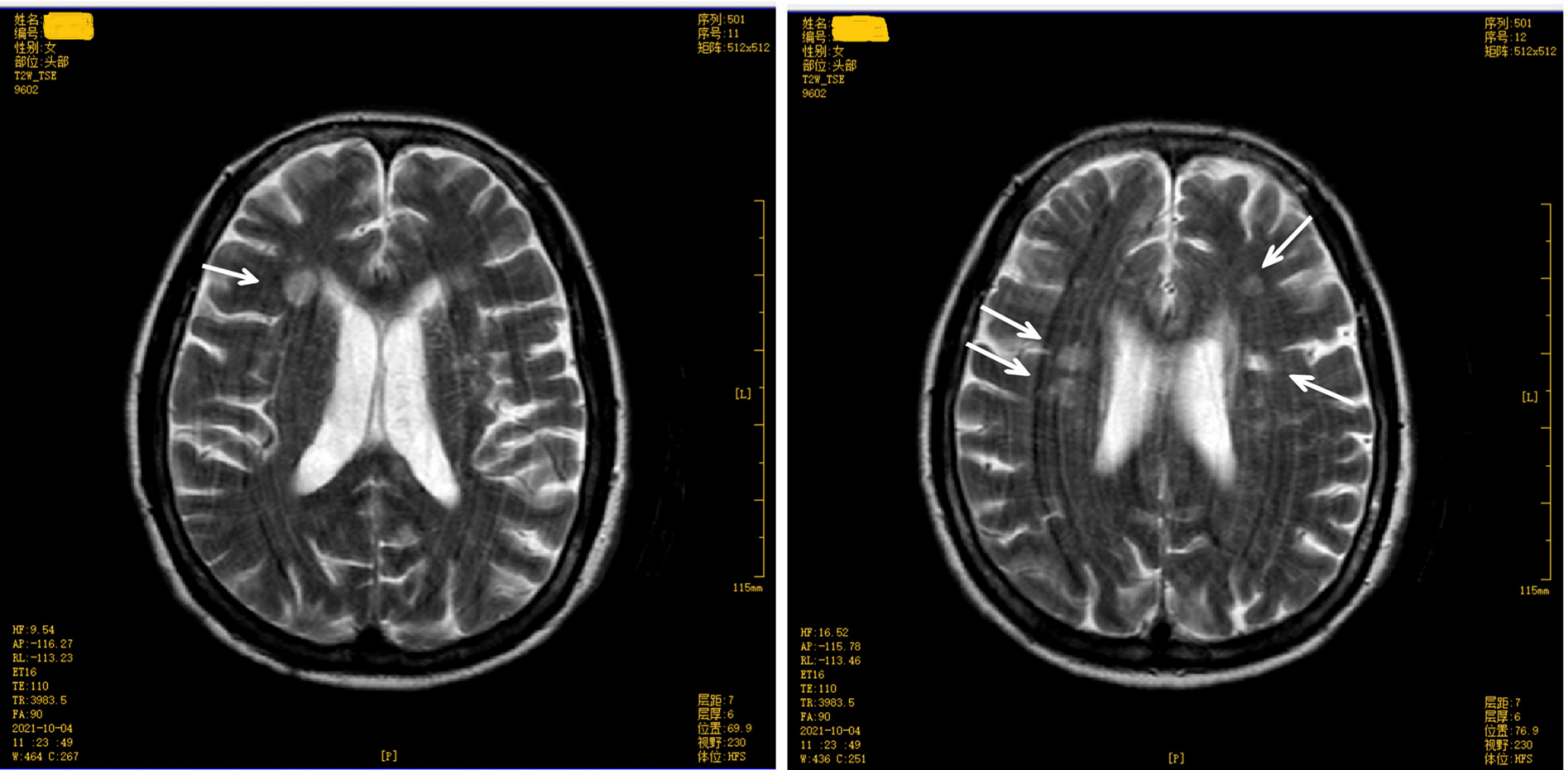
Brain magnetic resonance imaging (upper level of lateral ventricle) T2 weighted image shows multiple abnormal oval-shaped hyperintense signal lesions in the cerebral hemisphere and mesolobus.
